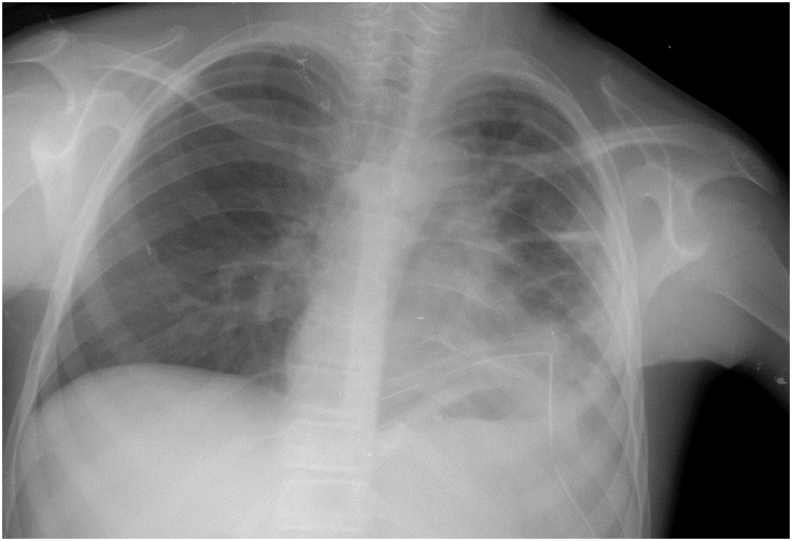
Patient's chest X-ray showing irregular opacity in the left lung field.
